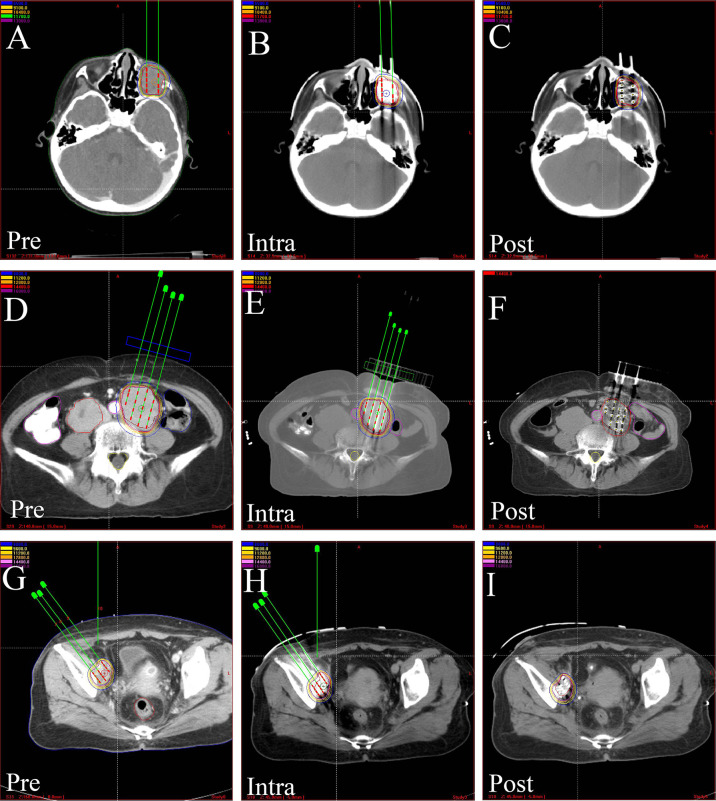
The CT-scan images of treatment plan assisted by 3D-PCT. Preoperative treatment plan, intraoperative verification, and postoperative dosimetric evaluation in, (A–C) orbital rhabdomyosarcoma, (D–F) kidney cancer, (G–I) pelvic recurrence of cervical cancer. 3D-PCT, three-dimensional printing coplanar template.
